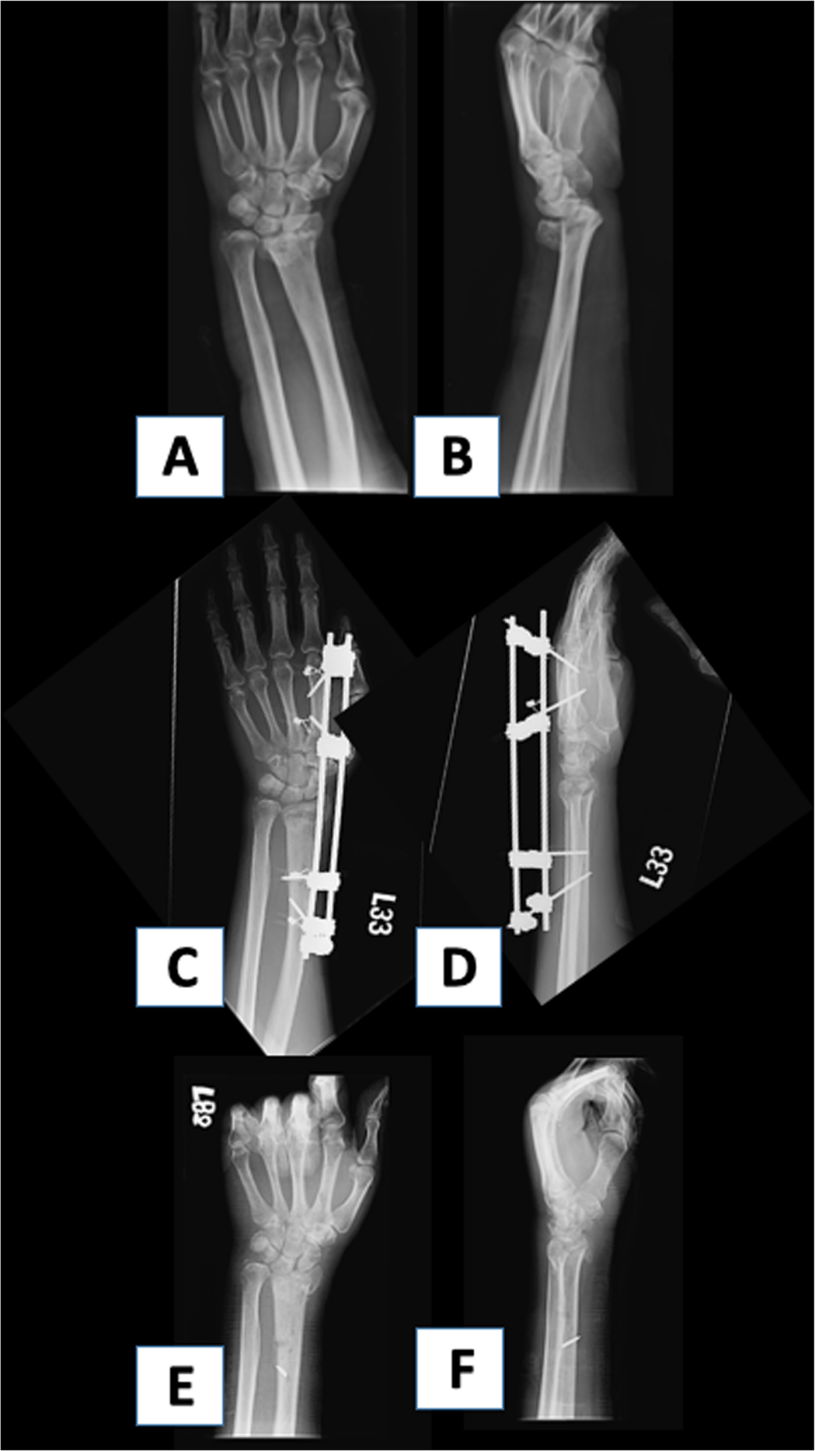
PD patient after ESF fixation. This was a 73-year-old female that presented with left distal radial fracture and fixed with ESF. a and b The preoperative radiographs. c and d Postoperative 3 days. e and f Postoperative 6 months status post removal of ESF with loss of reduction
